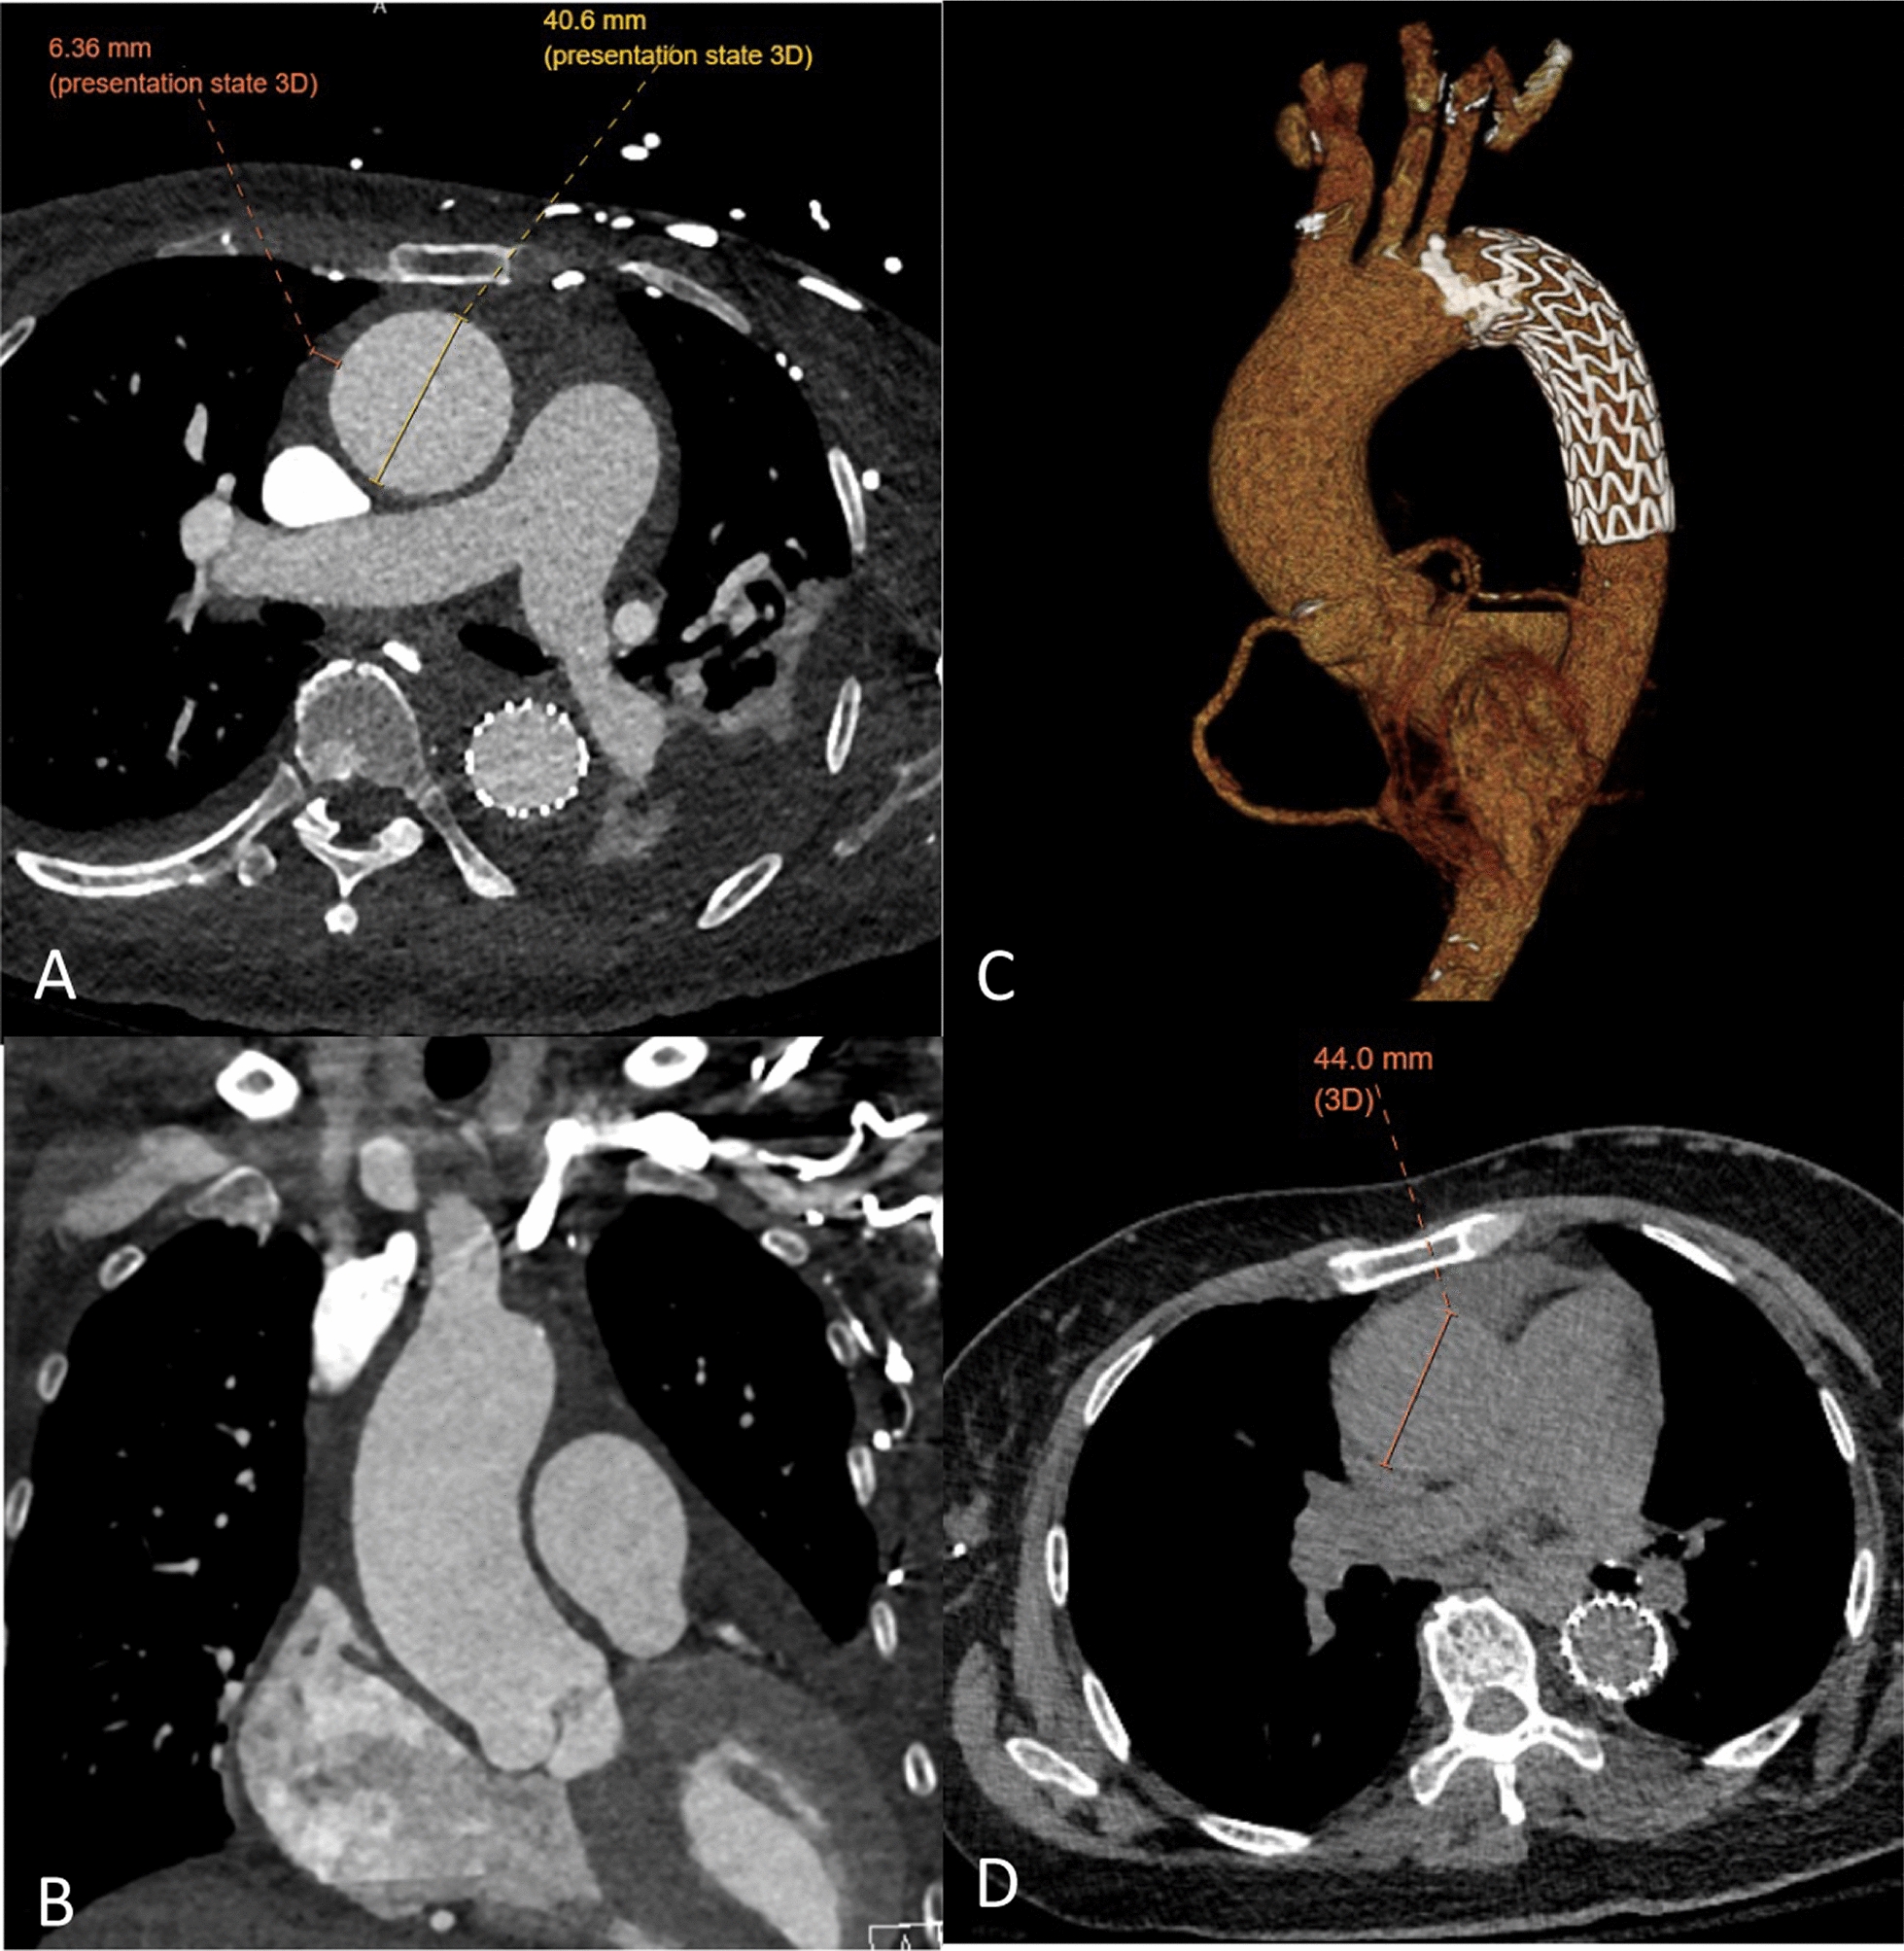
Post-operative Imaging. A, B, C 6 weeks after TEVAR. D Non-contrast CT 3 years after TEVAR
